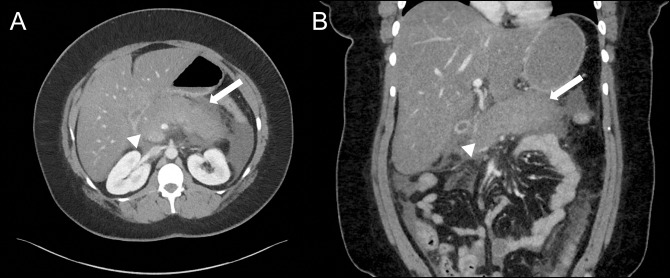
Abdominal and pelvic computed tomography with intravenous contrast in (A) axial and (B) coronal views showing the pancreas appearing diffusely edematous with surrounding stranding most severely affecting the body and tail with peripancreatic stranding/fluid (arrows) and sparing of pancreatic head (arrowheads). Biliary tree appears normal without choledocholithiasis and pancreatic duct is nondilated.
